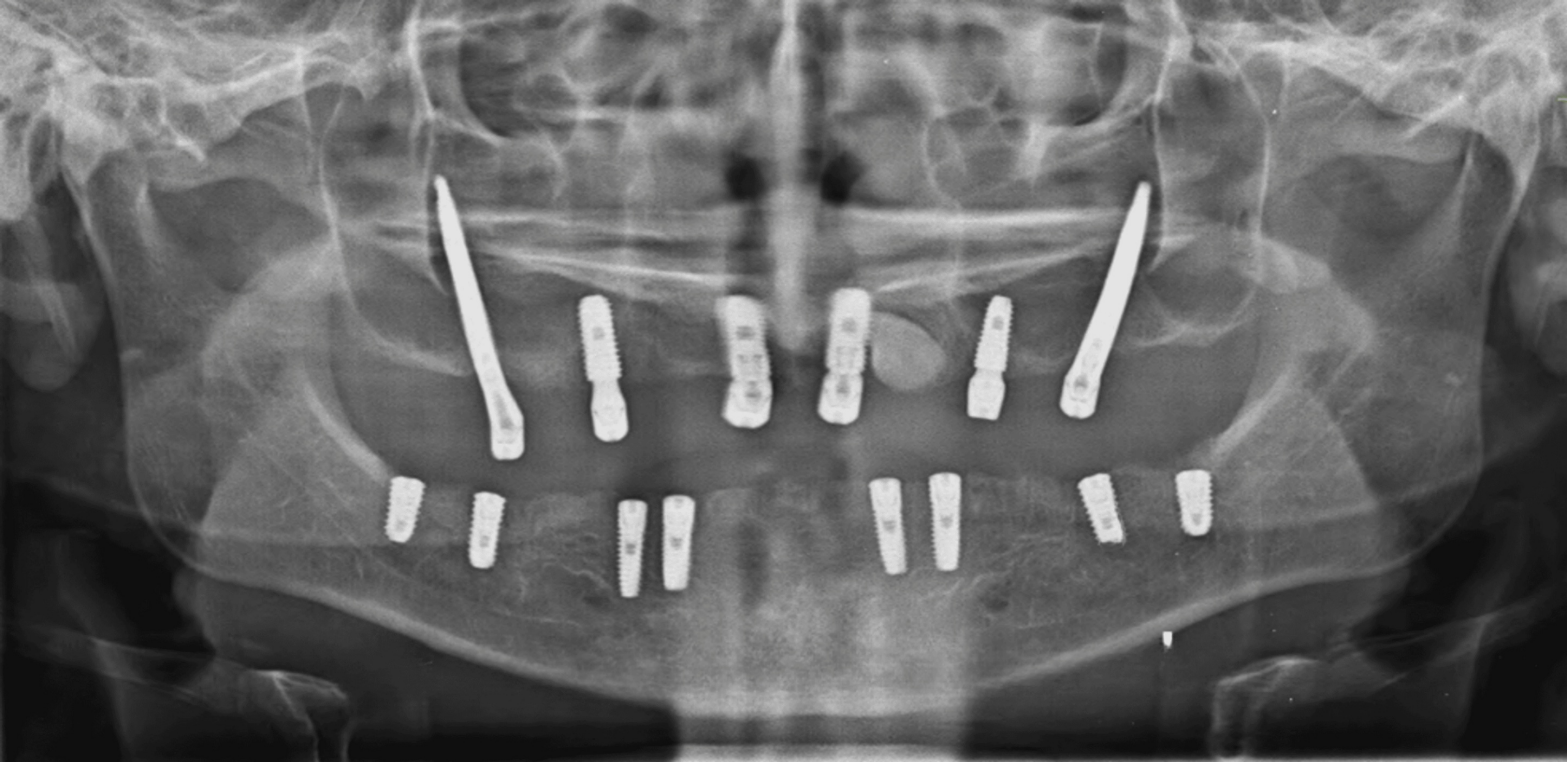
Postoperative OPG of the patient Image credit: Dr. Bhushan Mundada OPG: Orthopantomogram
